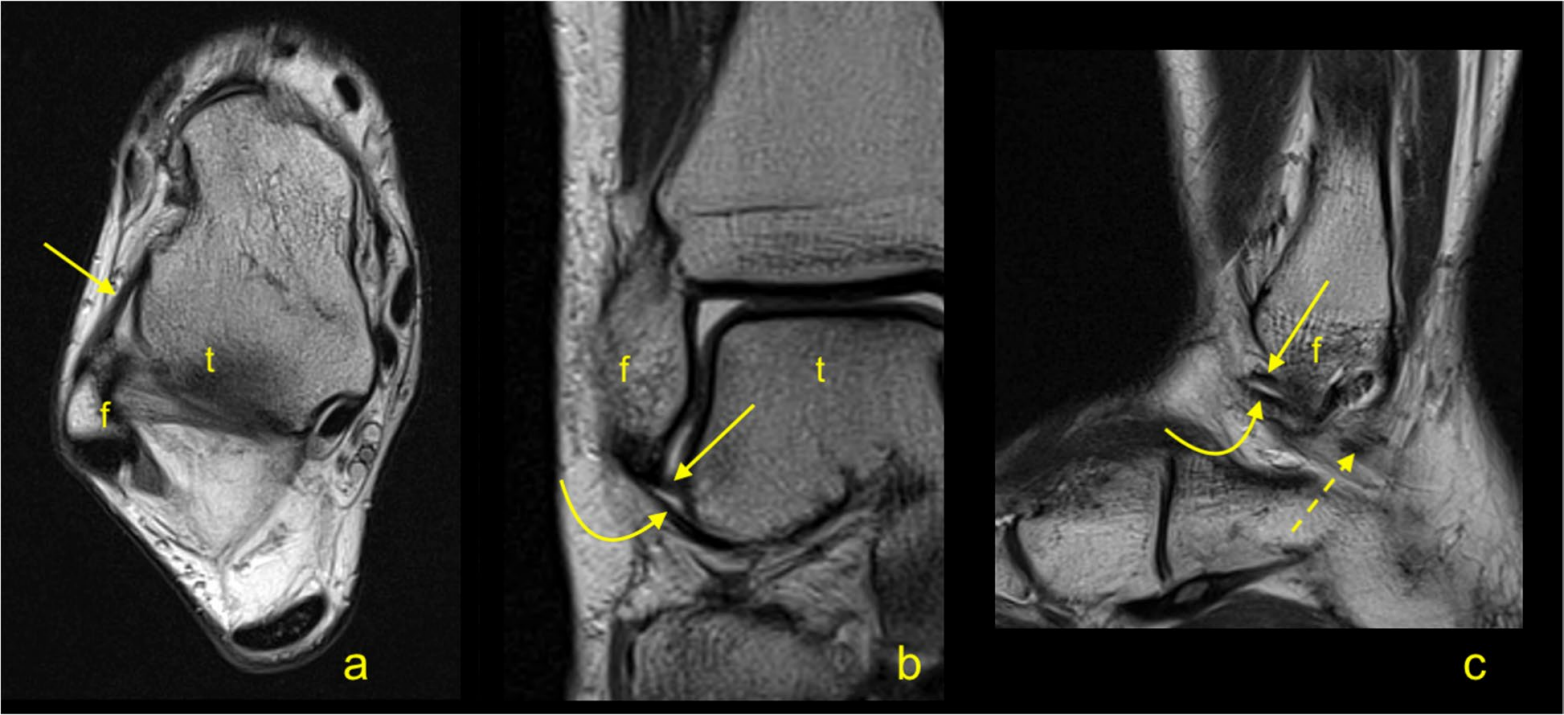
A double fascicular anterior talofibular ligament. Two asymmetrical fascicles are visible; the bigger one is located in a superior position (arrow), and the smaller one inferior (curved arrow). A T2-weighted sagittal section; b and c T2-weighted axial section; d and e T2-weighted coronal section. F, fibula; t, talus
